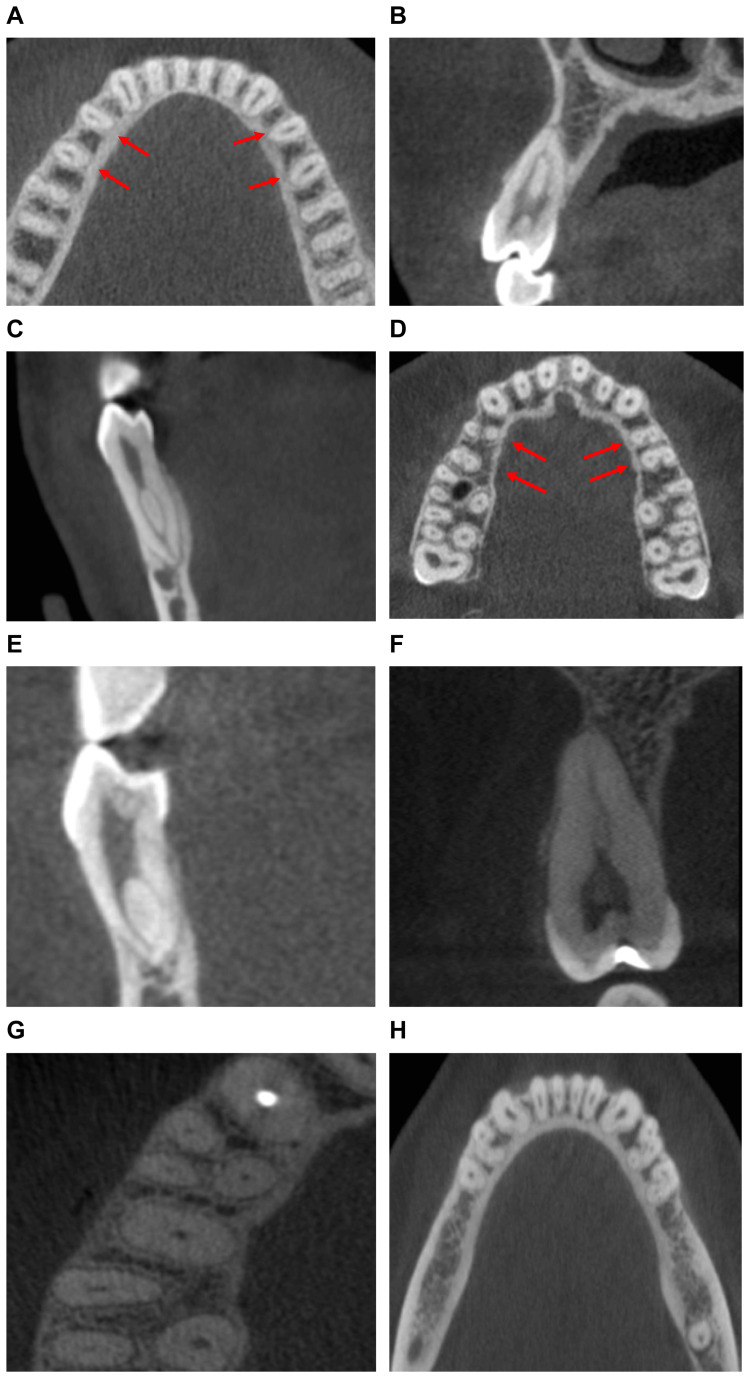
Root canal configuration of premolar teeth shown in CBCT images (A) Axial view showing type I canal configuration; (B) Coronal view showing type II canal configuration; (C) Coronal view showing type III canal configuration; (D) Axial view showing type IV canal configuration; (E) Coronal view showing type V canal configuration; (F) Coronal view showing type VII canal configuration; and (G, H) Axial views showing type VIII canal configuration. CBCT: cone-beam computed tomography
